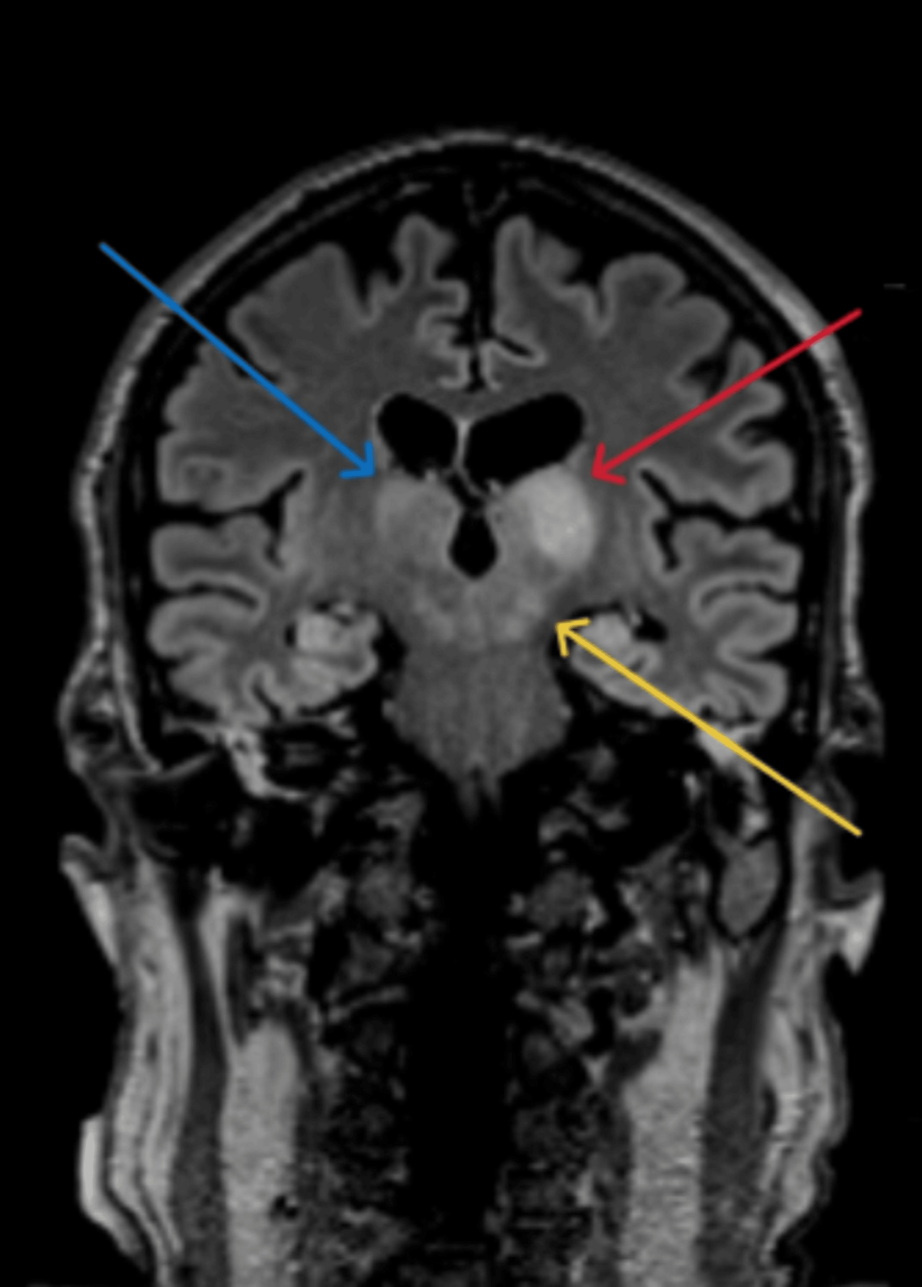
Coronal brain MRI showing increased FLAIR signal in the left thalamus (red arrow), lateral right thalamus (blue arrow), and midbrain (yellow arrow) MRI: Magnetic resonance imaging; FLAIR: fluid-attenuated inversion recovery
